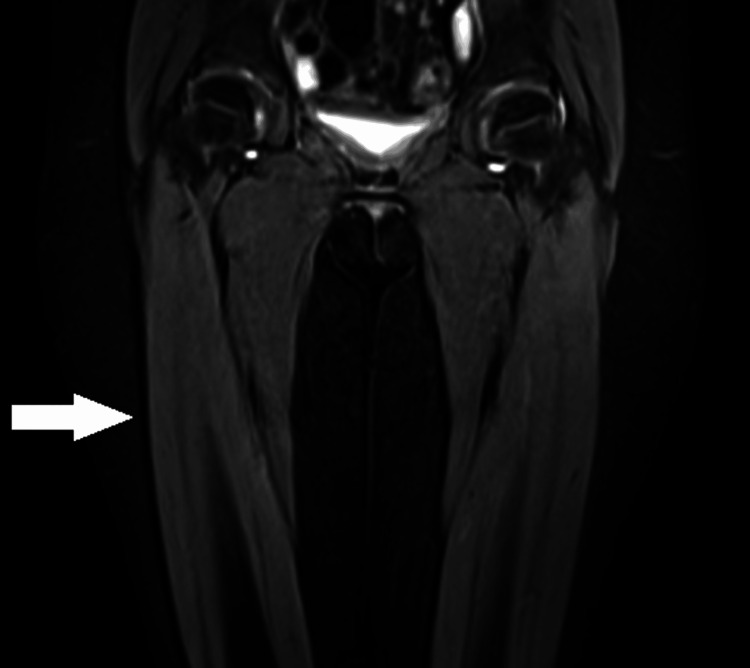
MRI of both lower limbs showing decreased muscle bulk in the right thigh
